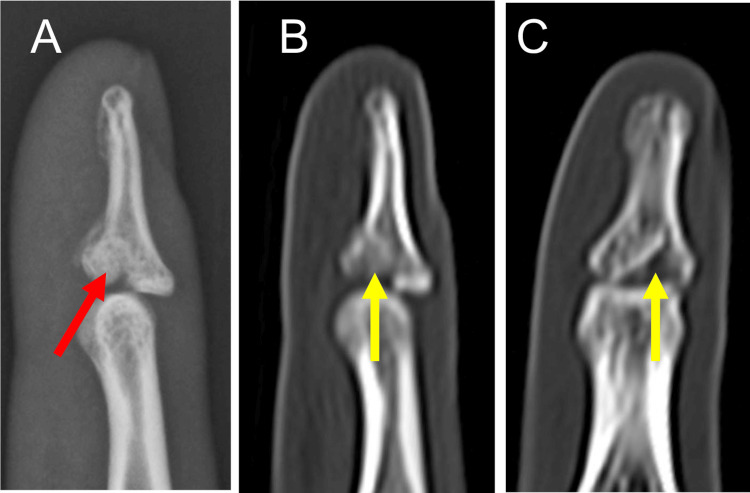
Preoperative radiograph and CT scans of Patient 2 The radiographs showing an impaction-type fracture (red arrow) in the lateral view of the right index finger (A). Sagittal view (B) and coronal view (C) show an impaction of the volar ulnar articular fragment (yellow arrows) and a dorsal subluxation of the DIP joint. DIP: distal interphalangeal
